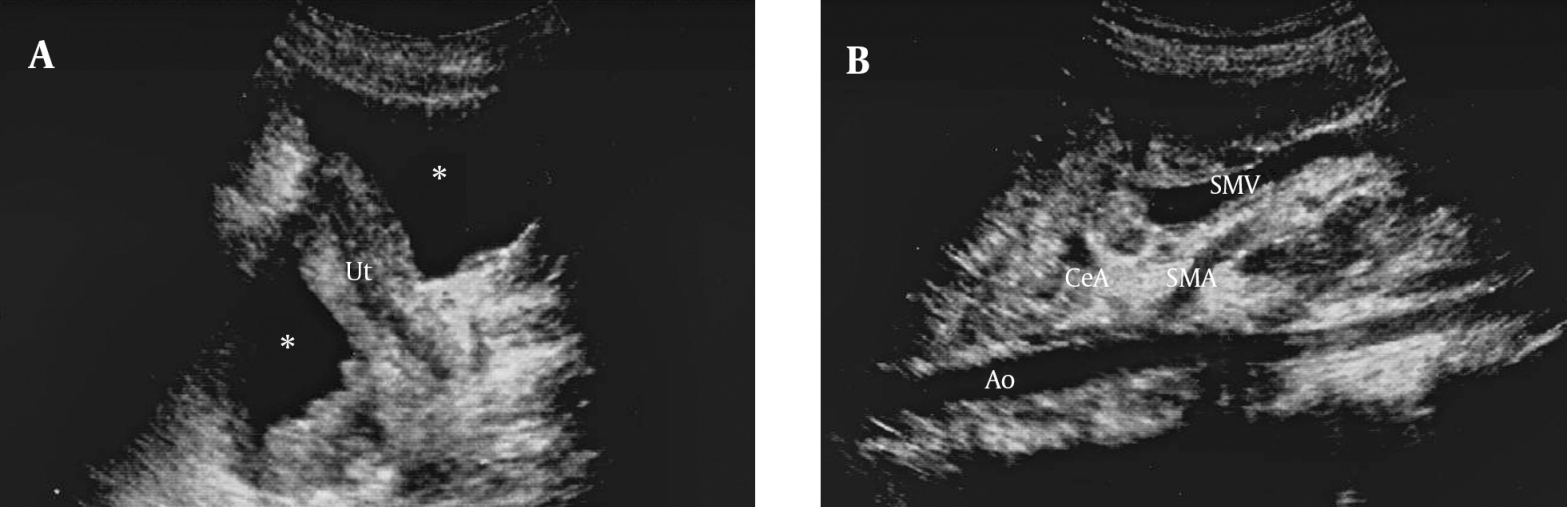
Abdominopelvic ultrasonography of the patient, A) Free fluid (*) in the pelvic cavity, Ut= uterus; B) Encasement of the celiac artery (CeA) and superior mesenteric artery (SMA) by mass lesion, Ao=aorta, SMV= superior mesenteric vein.
